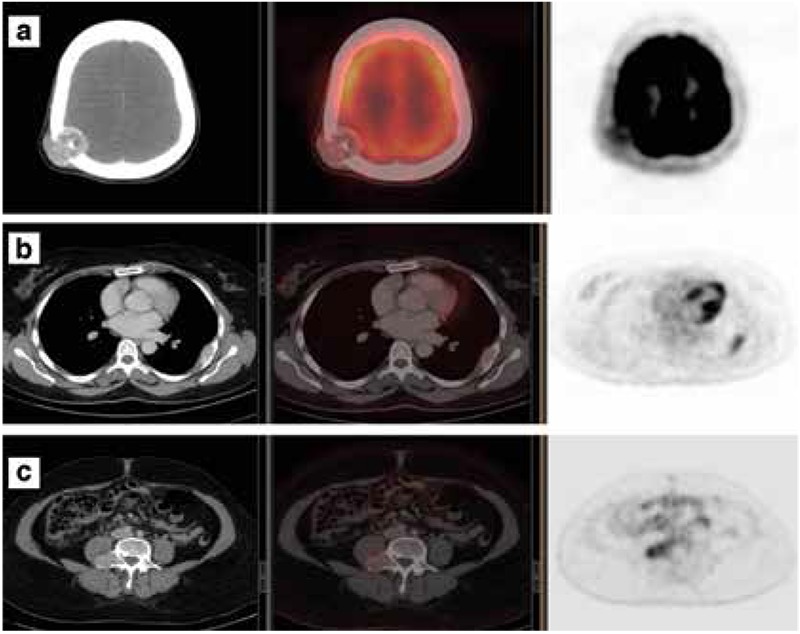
a) Trans-axial computed tomography, fusion positron emission tomography/computed tomography (PET/CT) and PET views of the skull showing metastatic deposit in the right parietal bone postero-laterally with interval progression in size and increase in both intracranial (extra-axial) and subcutaneous soft tissue components, b) Trans-axial computed tomography, fusion PET/CT and PET views of the chest showing a lytic lesion with a large soft tissue component in the 5th left rib postero-laterally which is mildly metabolically active (SUV_max_ of 3.4), c) Trans-axial CT, fusion PET/CT and PET views of the pelvis showing an expansile mass appreciable in the right pedicle and transverse process of the L4 vertebra without intra-spinal extension (SUV_max_ of 4.1)
